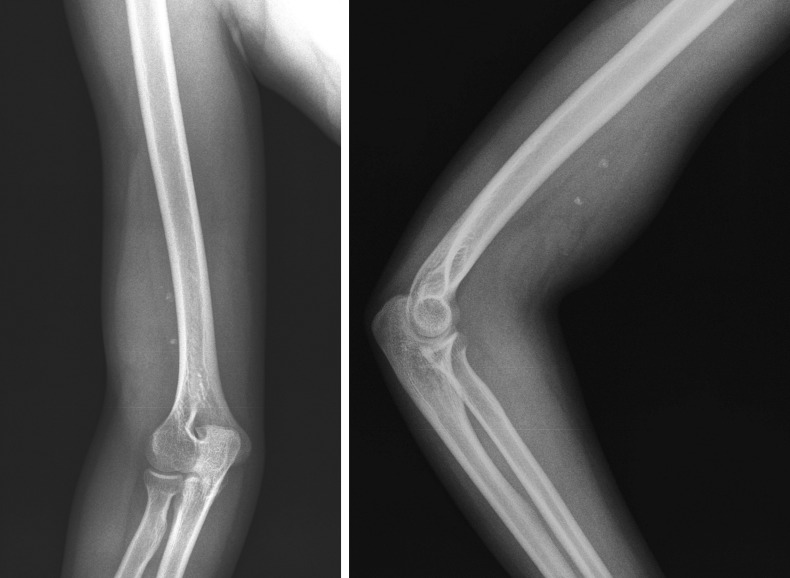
Plain upper limb X-ray in antero-posterior (a) and lateral (b) view showing a swelling of the lower third of soft tissues with calcification and intact bone structures
